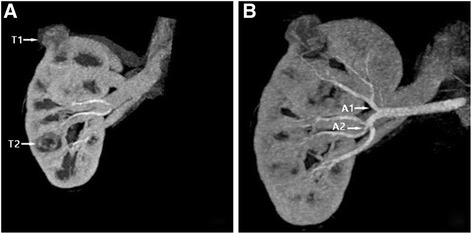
CTA was used to position tumors and their corresponding segmental arteries. a CTA showed the tumors in the upper (T1) and lower pole (T2) of right renal; b Superior, anterior and posterior branch were separated from the right renal artery near the renal hilum. Superior branch supplied the lesions in the upper pole (A1) and anterior branch supplied the lesions in the lower pole (A2)
